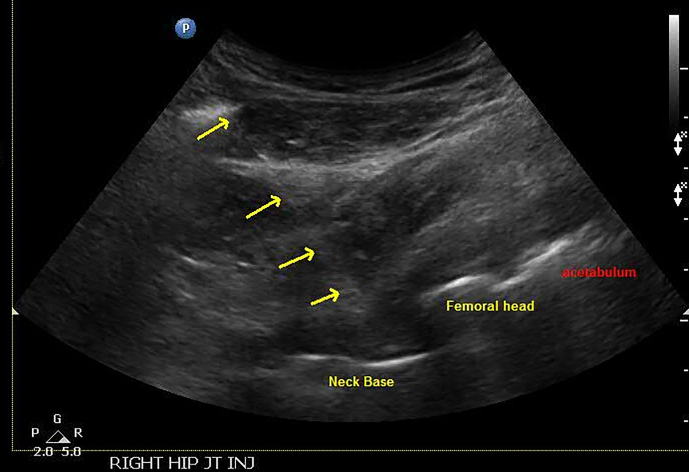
Ultrasound image with needle placement and labeled aspects of a
